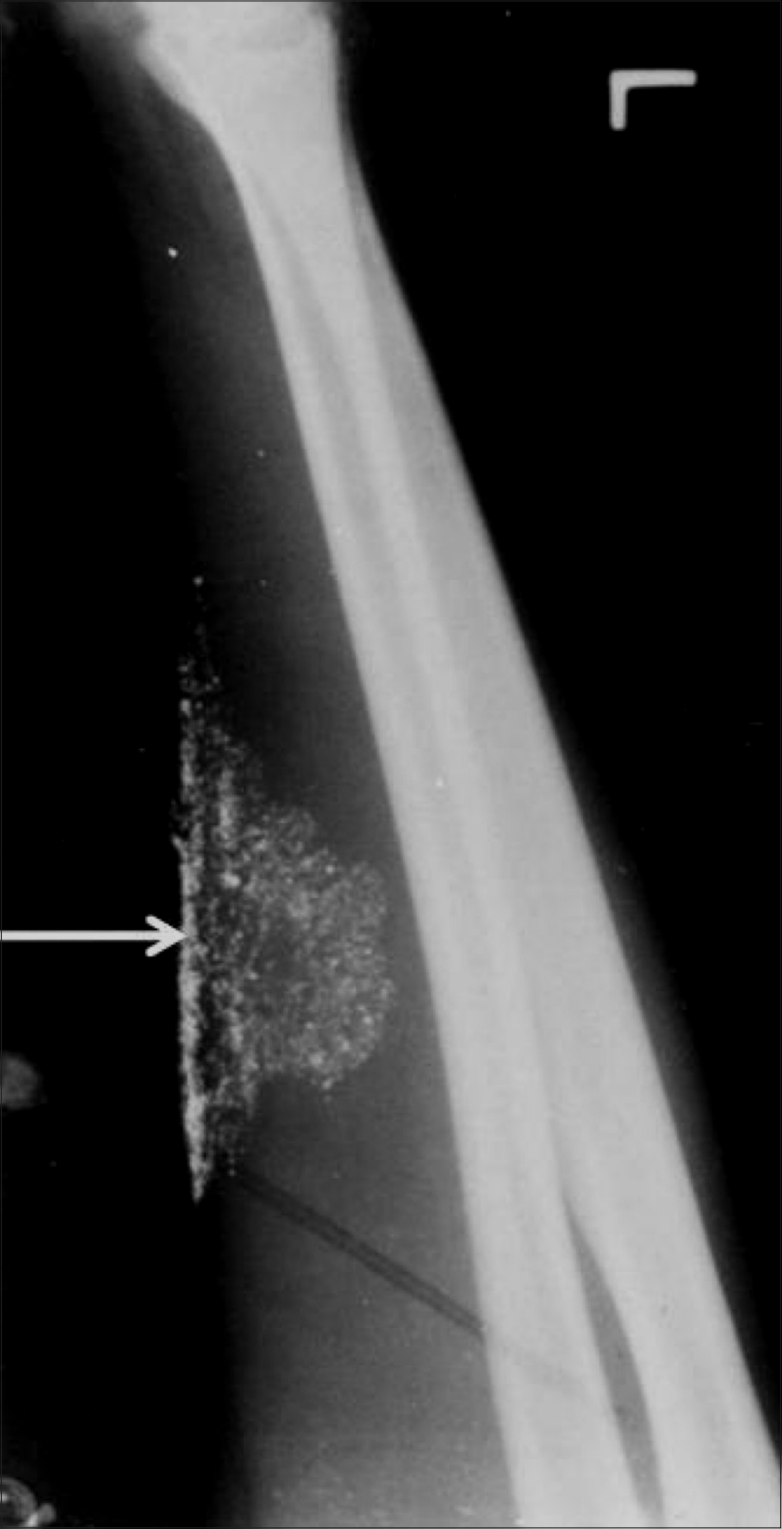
Oblique radiograph of the left forearm shows fine nodular opacities at the site of injection (arrow)
